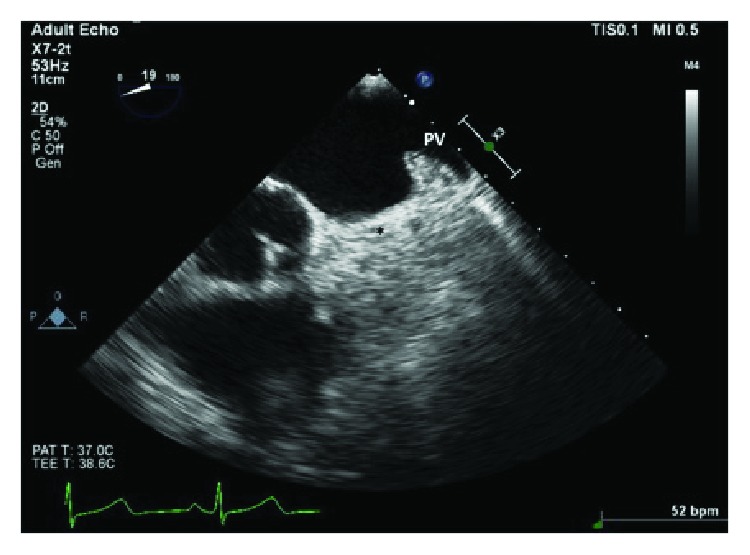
Transesophageal echocardiogram (TEE) at aortic valve level, 19-degree angle midesophageal view shows the pulmonary vein but the absence of the left atrial appendage at the usual anatomical location marked with an asterisk (^∗^).
